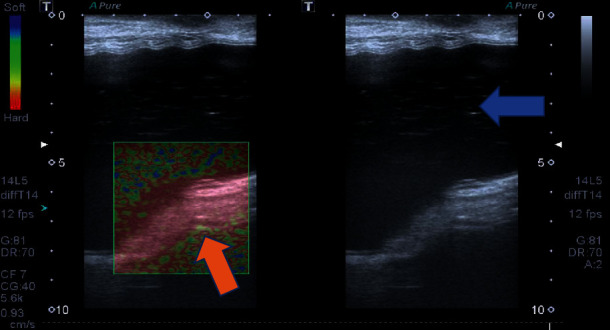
A 45-year-old patient with silicone implants for 7 years. Breast ultrasonography shows echotexture changes in the silicone implant contents (blue arrow) and an intracapsular mass associated with snowstorm artifacts, hard at elastography imaging compatible with silicone-induced granuloma of the breast implant capsule.
